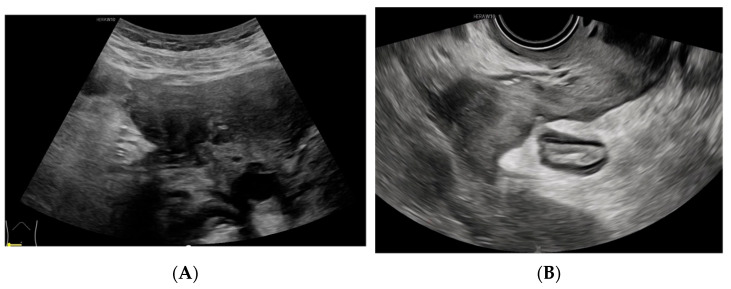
(A) A gradual reduction in the mass. (B) The eventual resolution of the mass, confirming a diagnosis of Actinomyces infection. Pelvic actinomycosis, a rare subacute to chronic granulomatous disease, constitutes approximately 3% of all actinomycosis cases [1]. Long-term IUD placement can lead to endometrial damage, facilitating the invasion of Actinomyces into the subendometrial layer. This invasion often extends to the fallopian tubes and ovaries, where Actinomyces secrete protein hydrolases that can exert a malignancy-like invasive effect and provoke an infection-like inflammatory response [2]. The clinical and imaging features of pelvic actinomycosis are not distinct, making its diagnosis challenging. Preoperative diagnosis is accurate in less than 10% of cases [3]. Although the imaging characteristics of pelvic actinomycosis are not unique, they also have some similar features with other diseases [2,4]. CT may reveal a thick-walled cystic or solid mass with heterogeneous enhancement. Ultrasound typically shows cystic–solid mixed echoes with abundant blood flow signals. In the early stages, the disease may present as an isolated mass. As the disease progresses, the mass enlarges, invading and compressing adjacent organs, and becomes increasingly irregular in shape and poorly demarcated from surrounding structures, complicating preoperative diagnosis. The affected intestinal wall may exhibit echogenic signs of inflammation due to invasion. The urinary tract can also demonstrate fluid dilatation due to compression and obstruction. On PET/CT, the mass often displays high fluorodeoxyglucose (FDG) uptake. Reflecting on this case, the localization of the lesion posed significant challenges. The copious inflammatory exudate and extensive adhesions within the pelvis obscured clear demarcation on tomographic images. Consequently, based on the patient’s prolonged gastrointestinal symptoms, both CT and PET-CT erroneously suggested an intestinal tract and mesentery origin. In contrast, ultrasonography, employing real-time scanning through various modalities and perspectives, accurately localized the lesion to the right adnexal region. Qualitatively, differentiating this disease from ovarian malignancy and pelvic tuberculosis is exceptionally challenging. Continuous differentiation throughout the course of the disease is imperative, especially considering the high failure rate (up to 50%) of Actinomyces cultures [1]. This necessitates extreme caution in postoperative pathological differentiation. Patients with ovarian cancer, predominantly older women, typically present with abdominal pain, distension, and cachexia, often lacking symptoms like chills and high fever. These cases are characterized by a marked elevation in CA125 levels. Unlike the extensive adhesions and sinus tract formation in the late stage of actinomycosis, larger ovarian tumors on imaging are often accompanied by significant abdominal or pelvic effusions and extensive lymphadenopathy. In this case, real-time dynamic ultrasound provided distinct advantages in determining the origin of the mass, assessing the extent of pelvic adhesions, and evaluating the involvement of surrounding tissues. It was also preferred for post-surgical efficacy assessment, playing a pivotal role throughout the diagnostic and treatment process.
